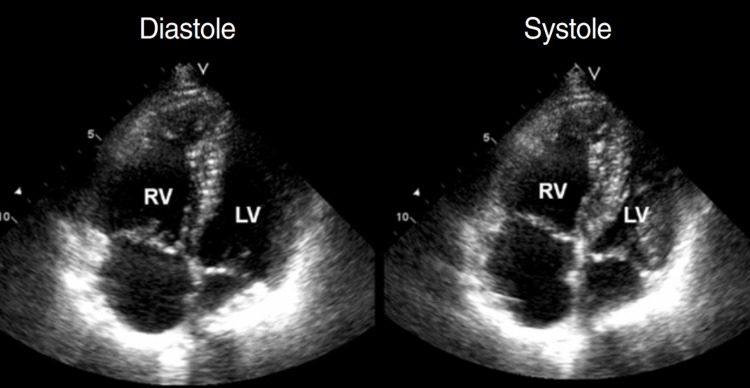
2D echocardiography of the patient The images show a dilated right atrium and right ventricle with suspicion of pulmonary embolism RV: right atrium; LV: left ventricle
